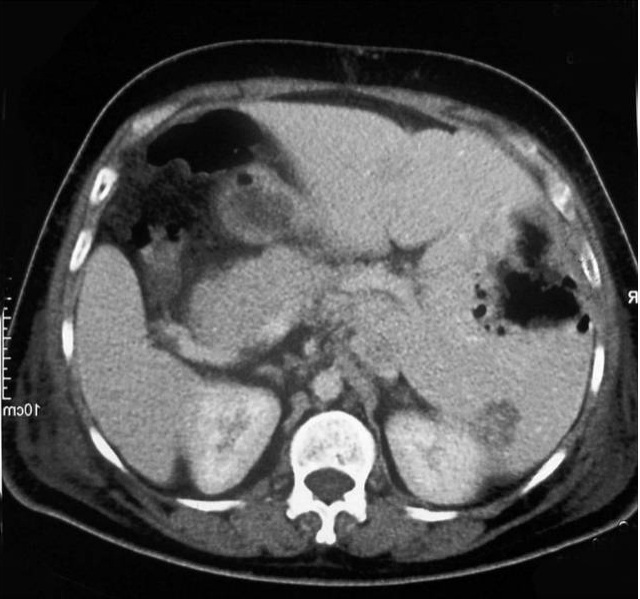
Abdominal CT showing multiple liver masses, largest one being 11 cm × 8.6 cm with abscess formation.
